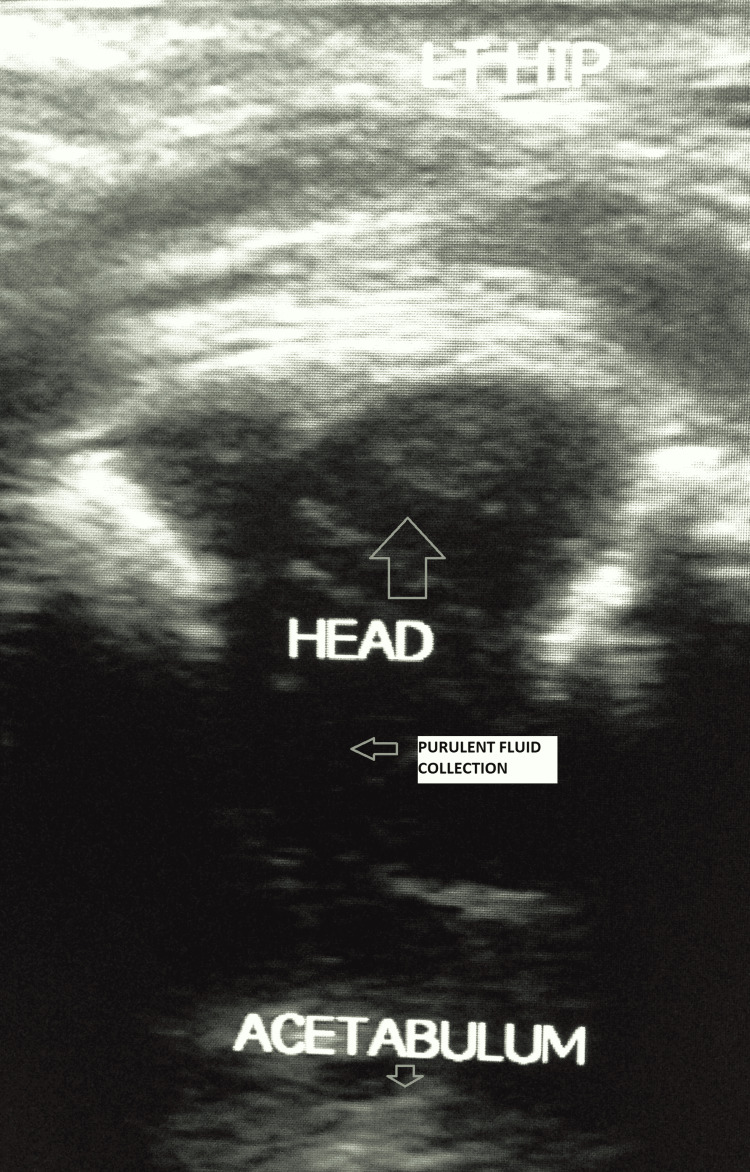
Ultrasound image of the neonatal hip in the axial view showing significant purulent fluid collection in the joint space LT HIP - LEFT HIP
